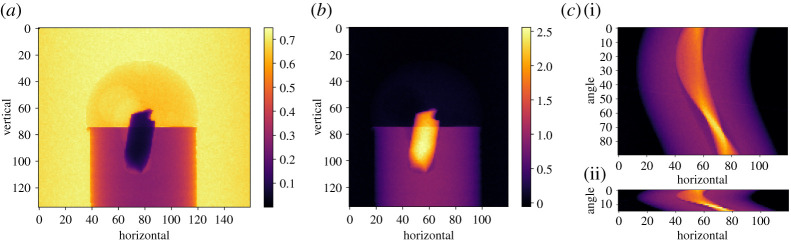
Raw and preprocessed three-dimensional parallel-beam X-ray CT steel-wire dataset. (*a*) Raw transmission projection. (*b*) Scaled, cropped, centred and negative-log transformed projection. (*c*)(i) Sinogram for slice vertical=103, all 90 angles. (*c*)(ii) Same, subsampled to 15 equi-spaced angles.
